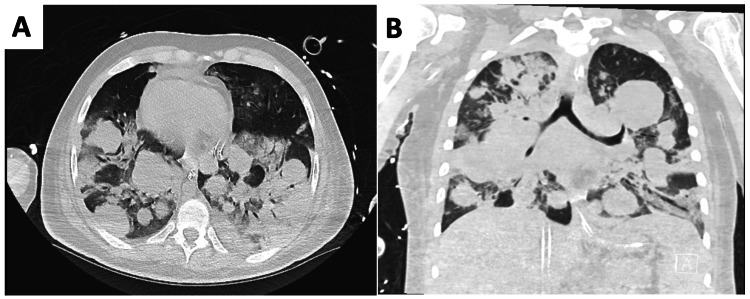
Axial (A) and coronal (B) chest CT images without contrast demonstrating extent of metastatic pulmonary disease from primary testicular non-seminomatous germ cell tumor. Both images show extensive solid nodules and masses throughout the lungs.
